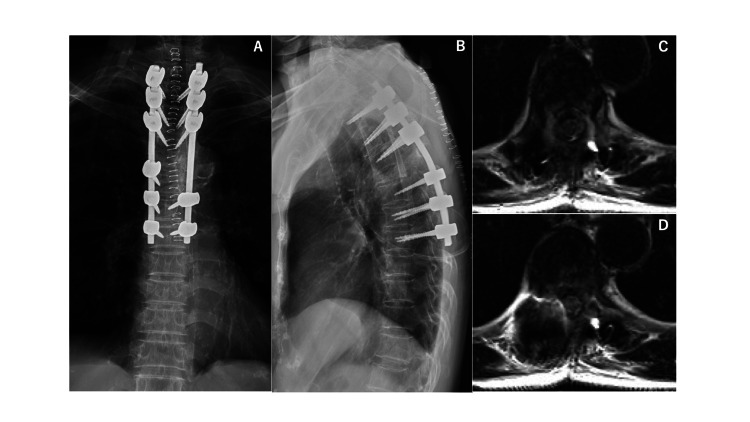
X-ray photographs and magnetic resonance images after the first surgery Frontal view (A) and lateral view (B) of X-ray images show good posterolateral fixation by screws and rods. After surgery, spinal compression by tumor is improved at the Th5 level (C, D).
